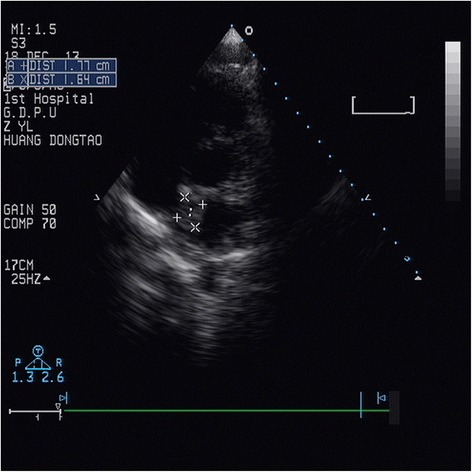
Transesophageal echocardiography demonstrating a myxoma of 1.9 × 1.2 cm rising from the left atrium
